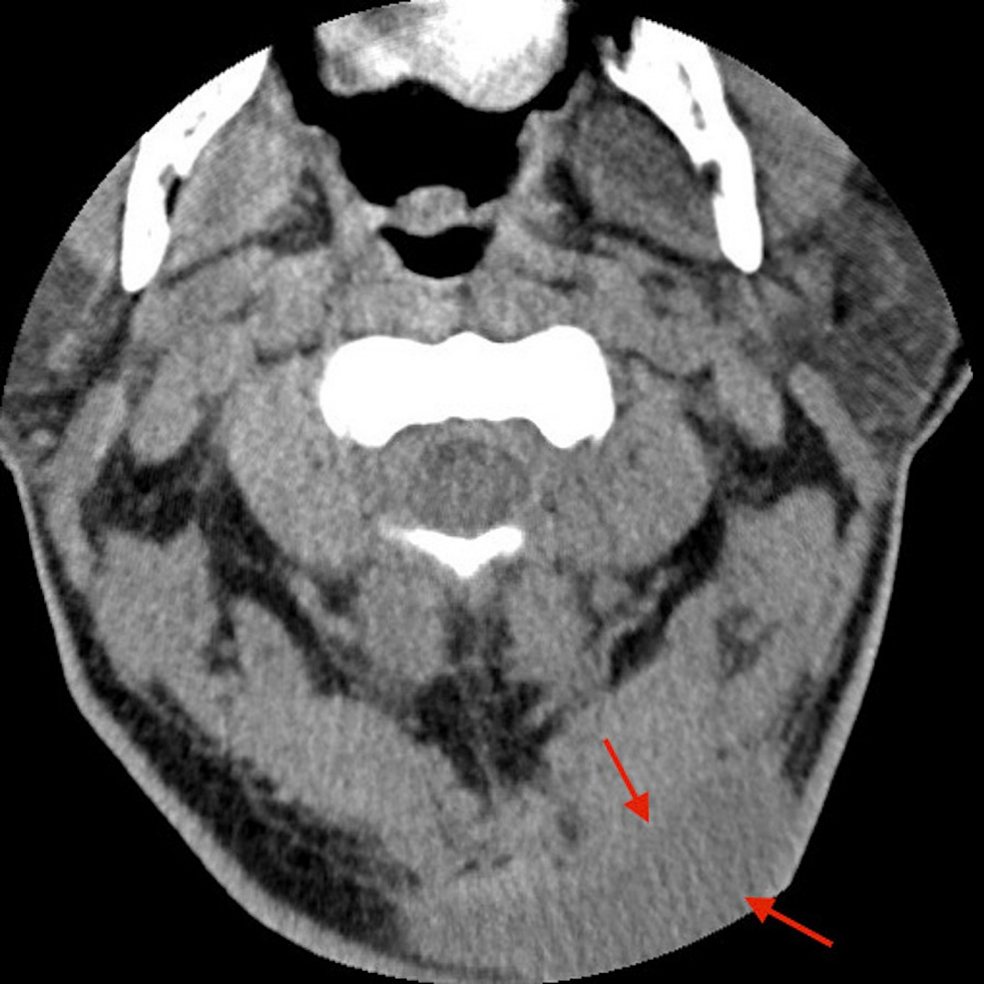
CT cervical spine without contrast showing large abnormality in the posterior subcutaneous region of the upper cervical spine at the midline and towards the left (arrows) CT: computed tomography
